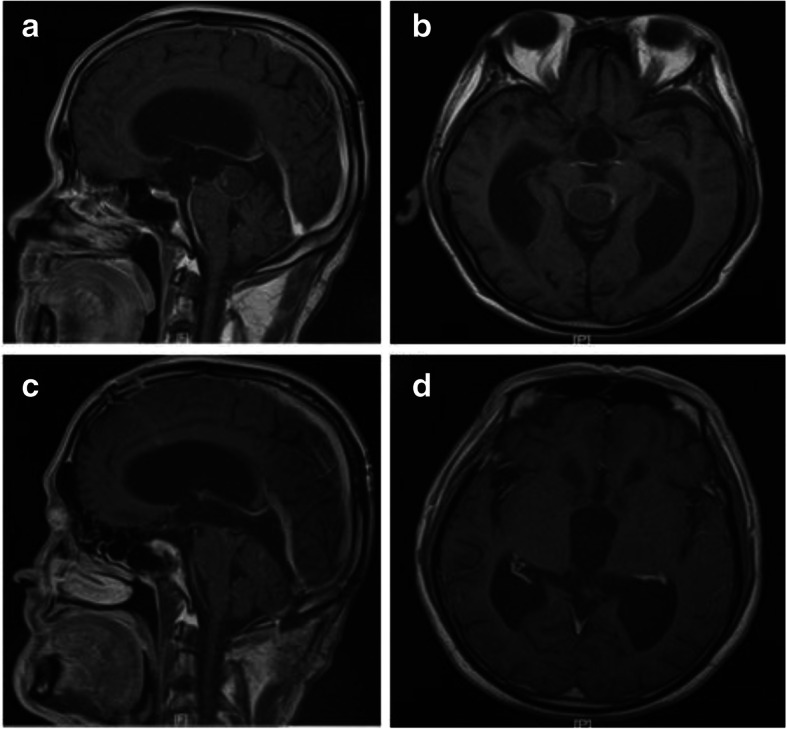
Preoperative and postoperative images of cavernous hemangioma: a and b preoperative MRI shows tumor in the pineal region; c and d One month after total resection of the tumor
